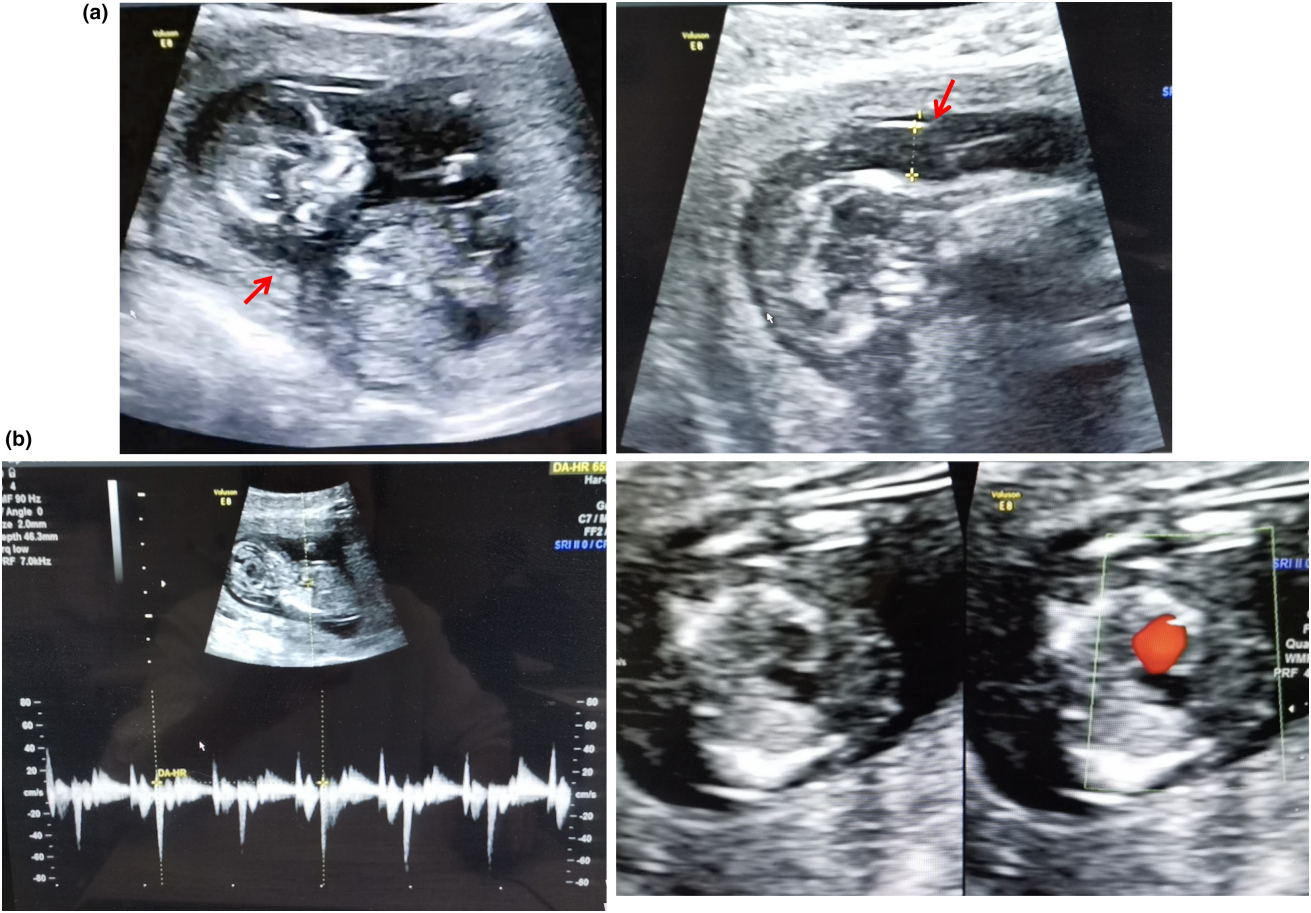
Clinical presentation of the fetus with *ASAH1*‐related disorder. (a) First trimester ultrasound showing increased NT thickness 6.7 mm (red arrow), severe hydrops fetalis, and cardiac anomalies. (b) Doppler ultrasound showed arrhythmia, bradycardia, and the ductus venosus alpha wave. The red arrow indicates the NT.
